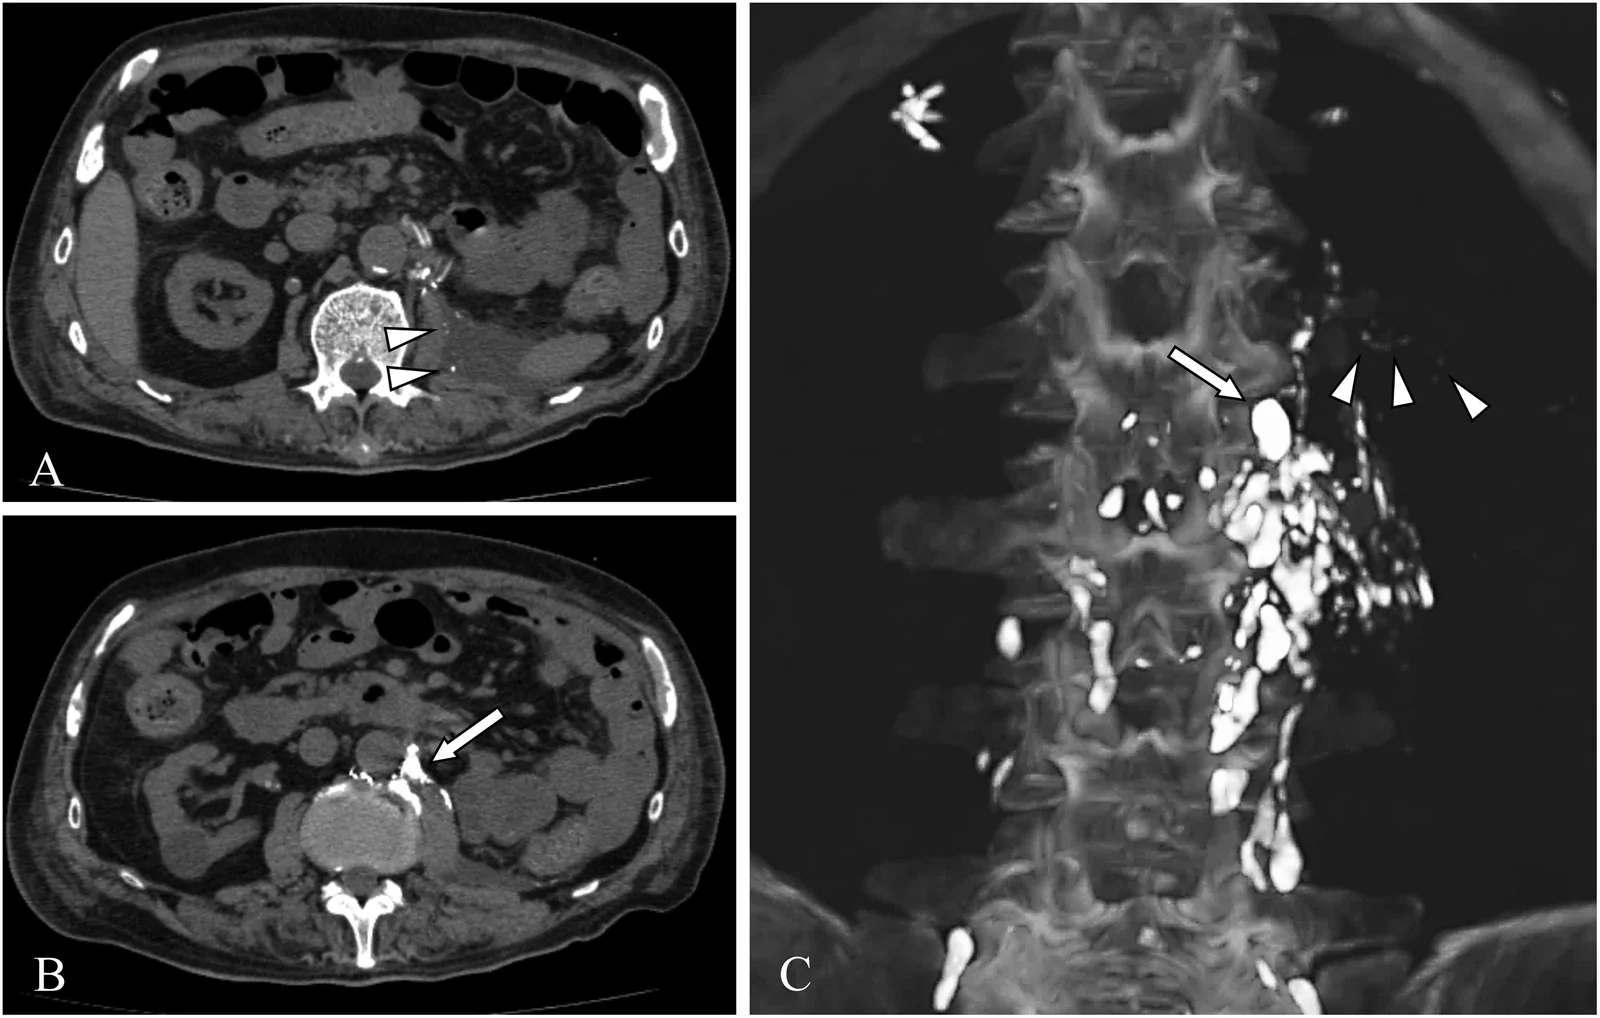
Noncontrast CT performed the day after transnodal lymphangiography. (A) Deposition of ethiodized oil in the retroperitoneal fluid collection near the nephrectomy site (arrowheads). (B) A lymphopseudoaneurysm is observed in the para-aortic region adjacent to the surgical site (arrow). (C) Maximum intensity projection image in the coronal view (bone window) shows a lymphopseudoaneurysm (arrow) and the leakage site (arrowheads).
